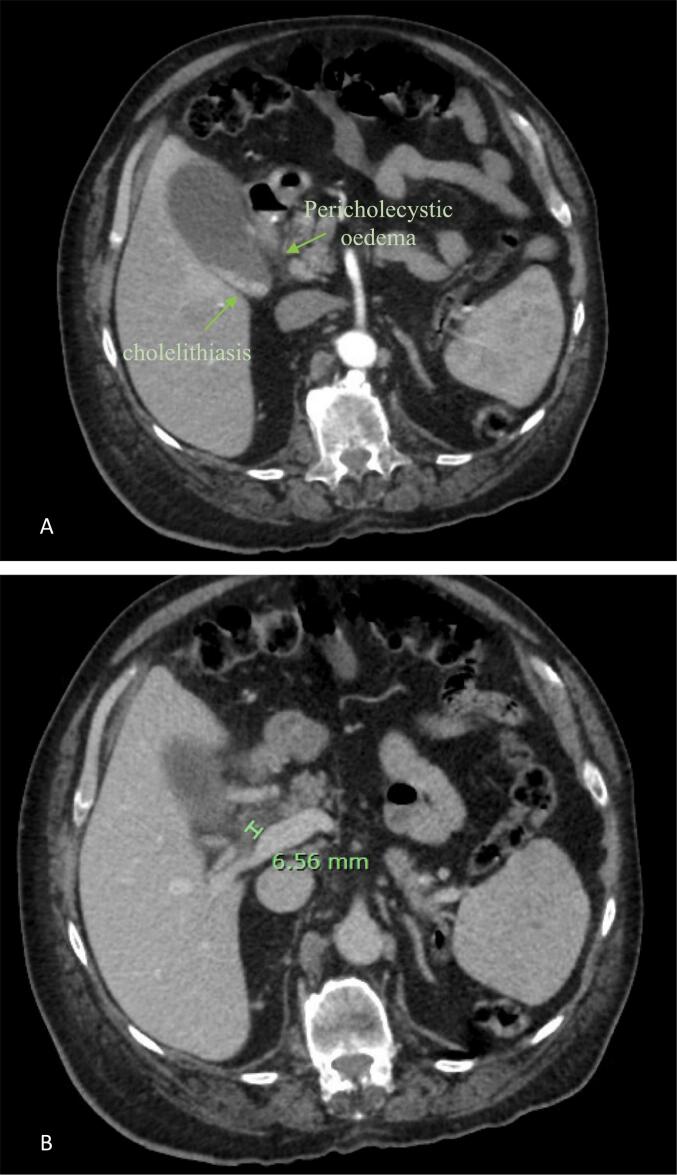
Axial section on Computed Tomography of Abdomen Pelvis (CTAP) showing cholelithiasis, peri-cholecystic oedema (A) and common bile duct diameter (B).
